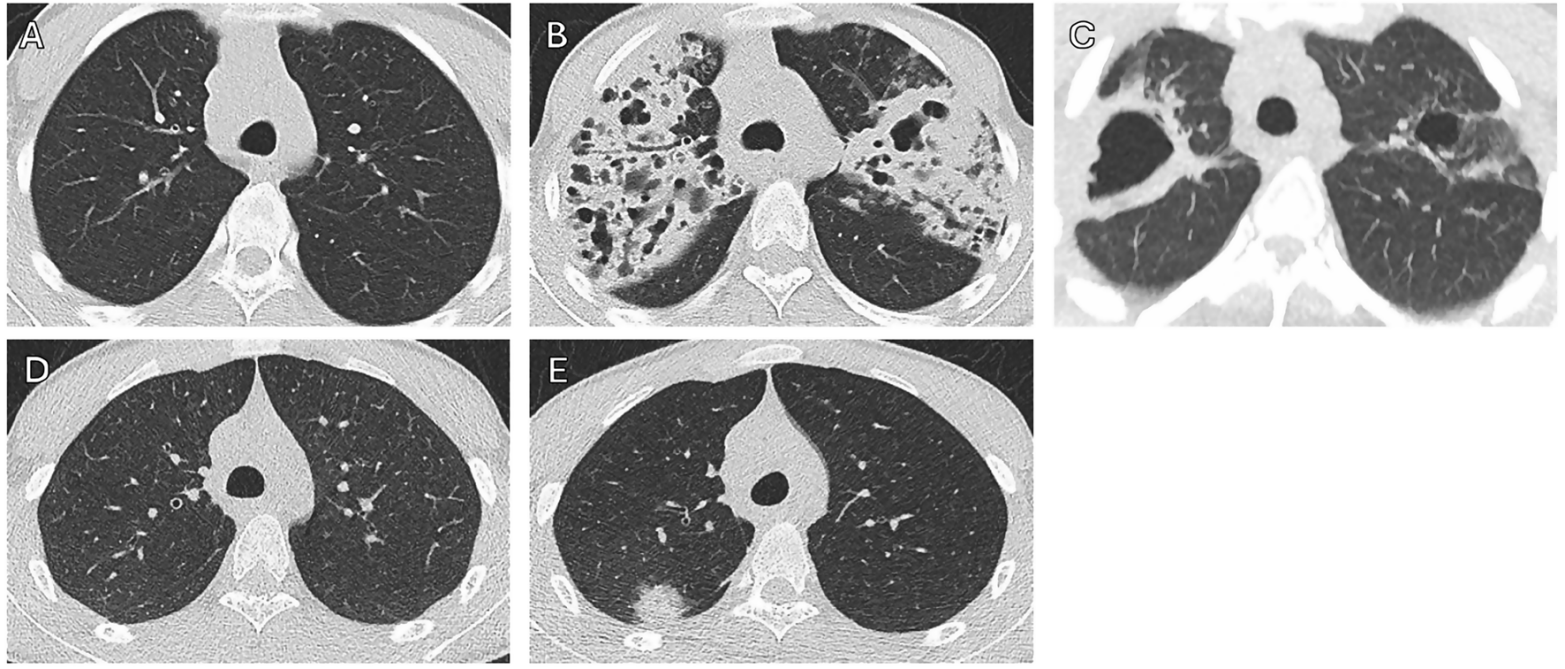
Serial chest CT scans of patients 7 and 8. Legend: A: Patient 7 in August 2020; B: Patient 7 in March 2021; C: Patient 7 in January 2024; C: Patient 8 in August 2020; D: Patient 8 in March 2021
